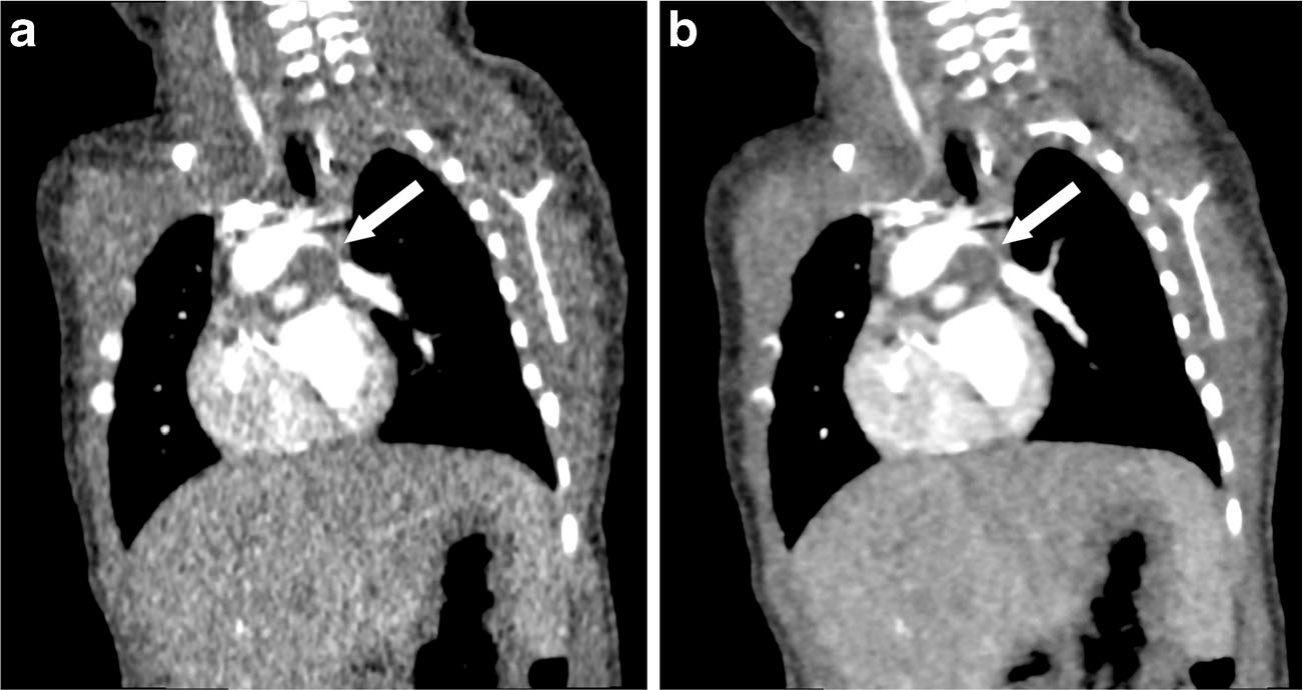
Coronal contrast-enhanced CT images in a 7-day-old boy with a double-outlet right ventricle, aortic origin of the left pulmonary artery, severe left pulmonary artery stenosis, ventricular septal defect and atrial septal defect. a Hybrid iterative reconstruction. b Full iterative reconstruction. Although left pulmonary artery stenosis (*arrow*) is brokenly depicted with hybrid iterative reconstruction and patency is ambiguous, patency is better demonstrated with full iterative reconstruction. The CT window settings of both figures are identical
